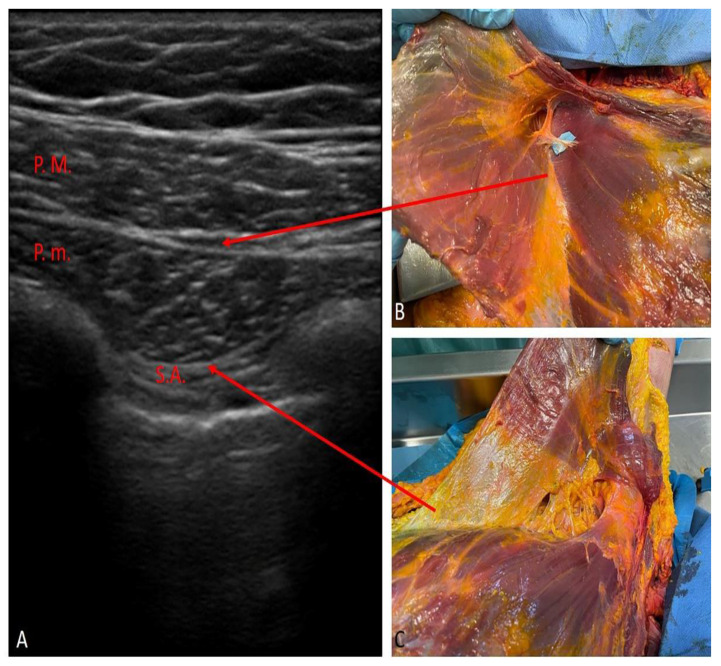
PECS II block: (A) oblique ultrasound imaging scan along the medioclavicular line 2nd rib level; P.M.—pectoralis major muscle; P.m.—pectoralis minor muscle; S.A.—serratus anterior muscle. (B,C) anatomical correlation dissections of the sites of inter-fascial injections.
